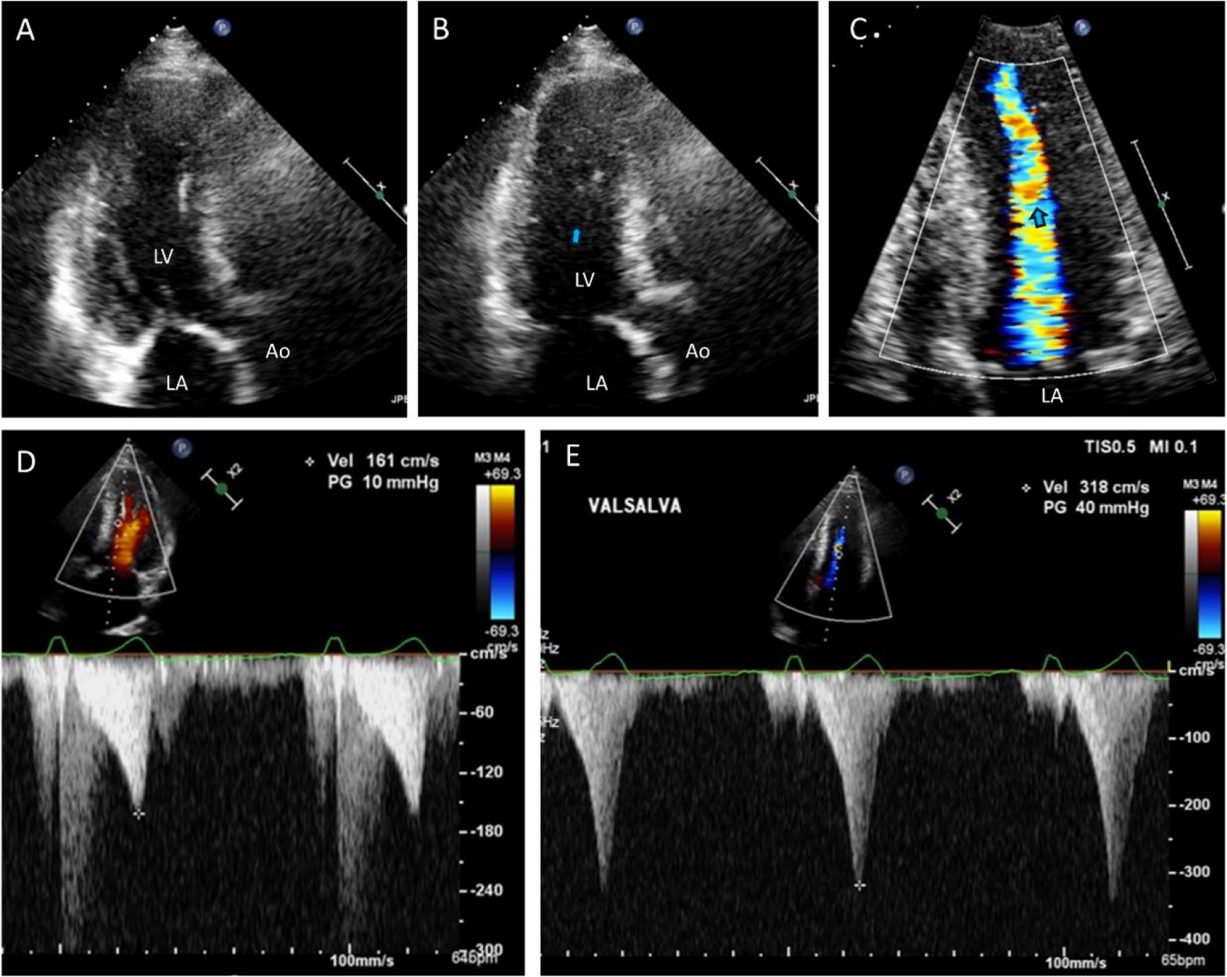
A representative case showing systolic intracavitary gradients after successful surgical aortic valve replacement. After successful aortic valve replacement, apical views show (A, B) strong contact between the hypertrophied papillary muscle and ventricular septal wall with (C) marked flow acceleration. Doppler tracing shows (D) typical dagger-shaped peak systolic velocity, which (E) intensifies with the Valsalva maneuver
